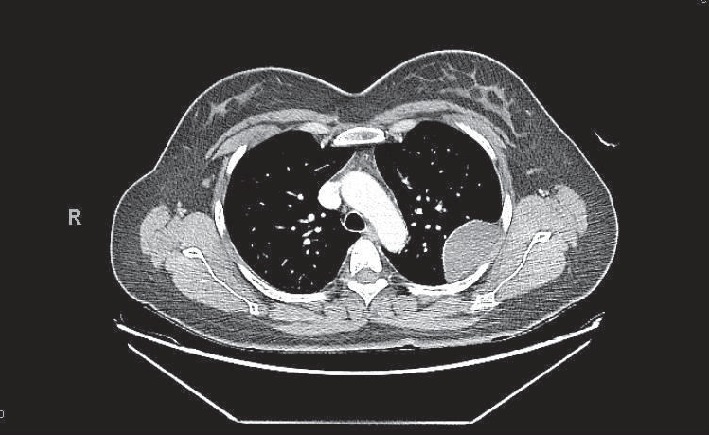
A crescent shaped, pleural based mass, measuring 6 × 4.9 × 3.8 cm, with some modelling and cortical irregularity of the superior aspect of the left lateral fourth rib as seen on CT.
